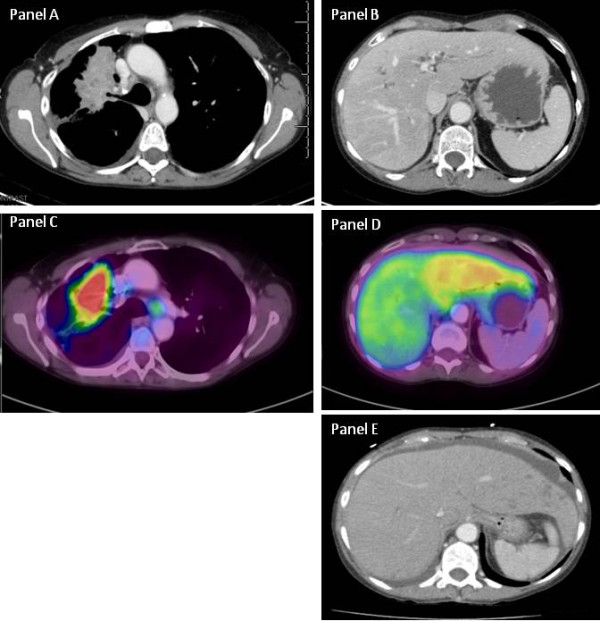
Case 2: Computed tomography (CT) and positron emission tomography. Panel A: Computed tomography of the chest shows the primary lesion in the right upper lobe caused by partial obstruction of the right upper lobe bronchus. Panel B: The CT scan of the liver shows essentially a normal-appearing liver without focal infiltrates present. Panel C: Positron emission tomography scan of the primary lesion shows intense fluorodeoxyglucose (FDG) uptake with some uptake noted in lymph nodes. Panel D: The right lobe of the liver looks normal, whereas the left lobe shows diffuse low FDG uptake suggestive of a pathological process. Panel E: A homogenous liver.
